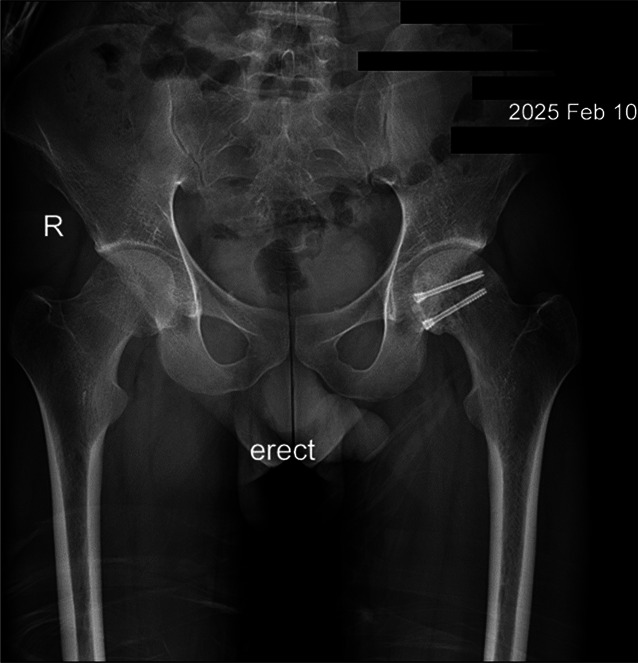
Two-year follow-up pelvic radiograph, showing the two Herbert screws used to fixate the left femoral head.
